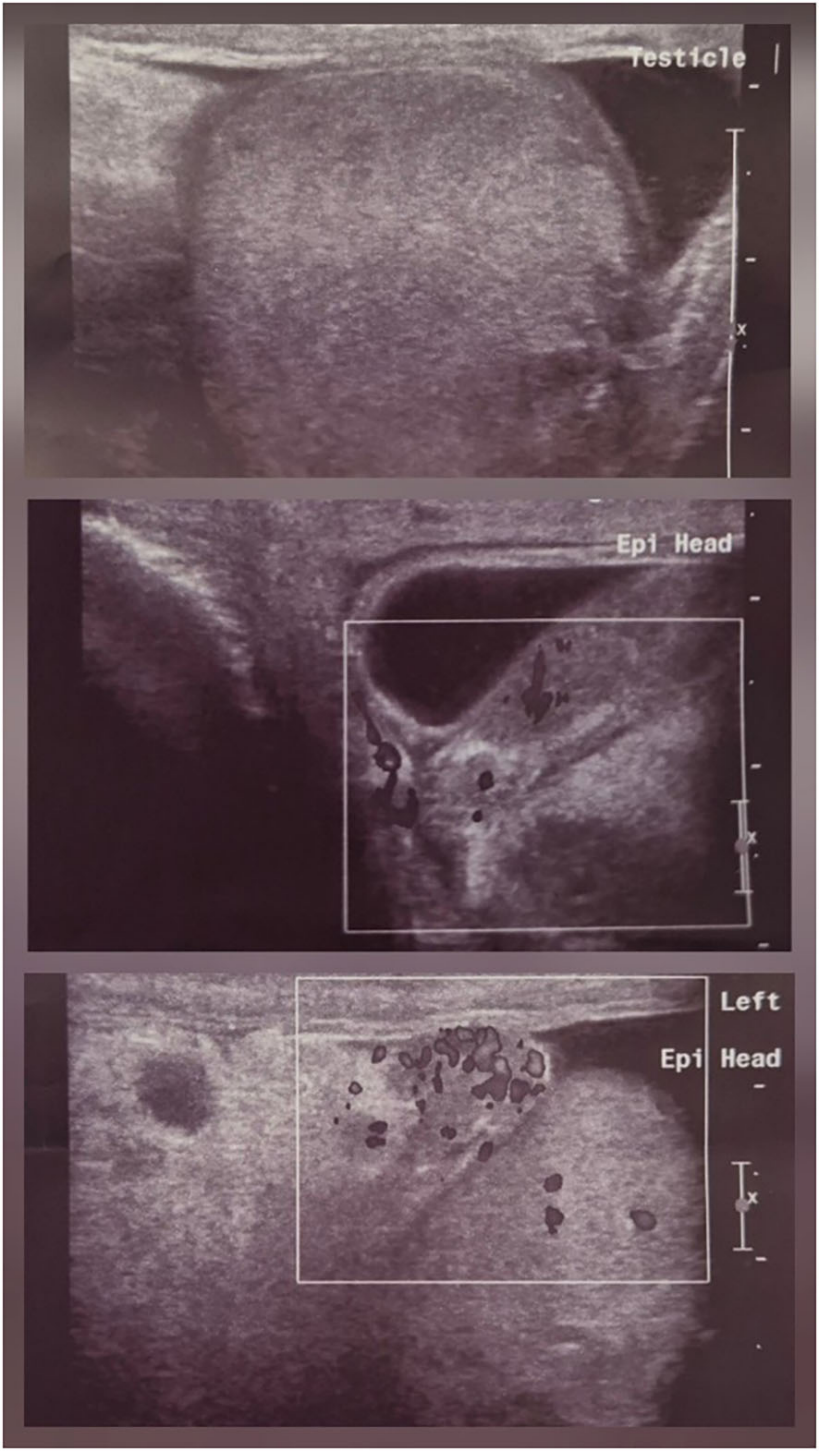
Case 2. Scrotal ultrasound. Enlarged, hypoechoic testis and epididymis with markedly increased vascularity.
